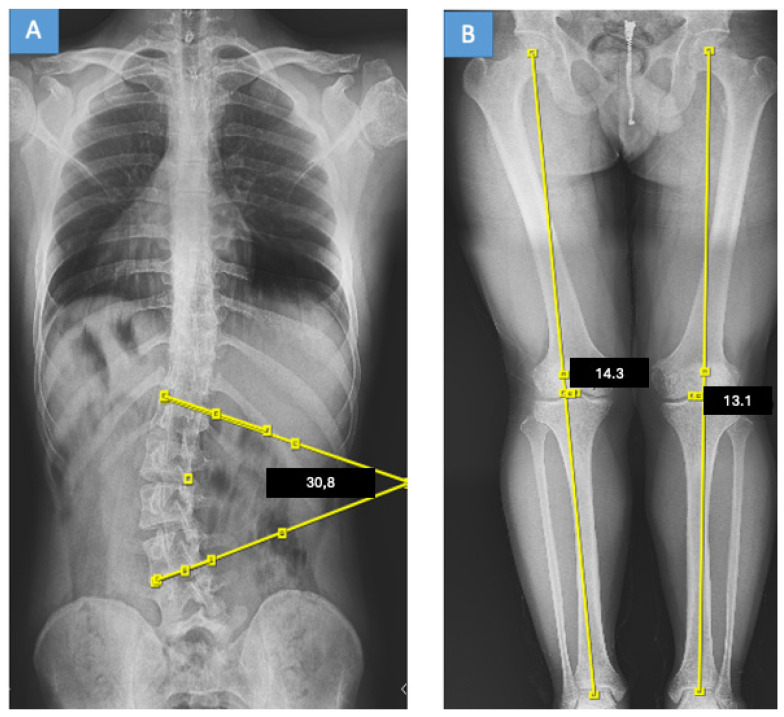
Representative radiographs of a patient with lumbar idiopathic scoliosis and a Lenke type 5C curve treated non-operatively with bracing. (A) Standing full-spine anteroposterior radiograph showing pelvic obliquity. (B) Full-length weight-bearing lower-limb radiograph showing mechanical-axis assessment; yellow lines indicate the mechanical axes, and marked points indicate reference landmarks for MAD measurement.
